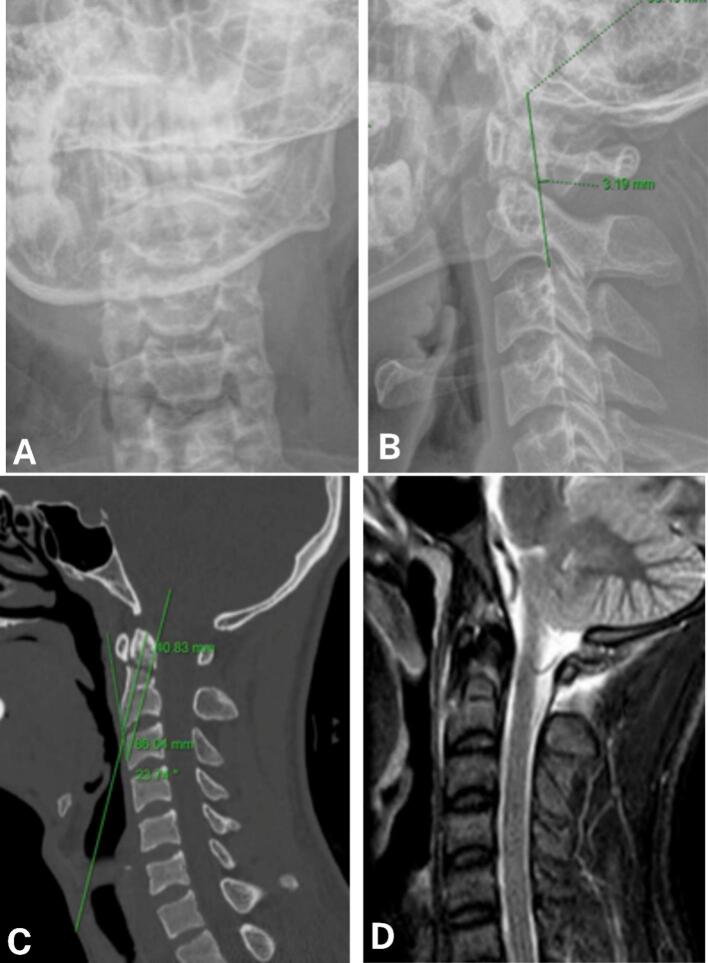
Preoperative X-ray and CT scan images of C2 odontoid process fracture type II. A, B: X-ray images depicting C2 odontoid process fracture type II. C, D: MRI images depicting C2 odontoid process fracture type II. B, C: bone entry point; estimated screw angle 24° to the longitudinal axis; screw length 40 mm; distance from the anterior neck skin surface to the C2 body is 86 mm.
